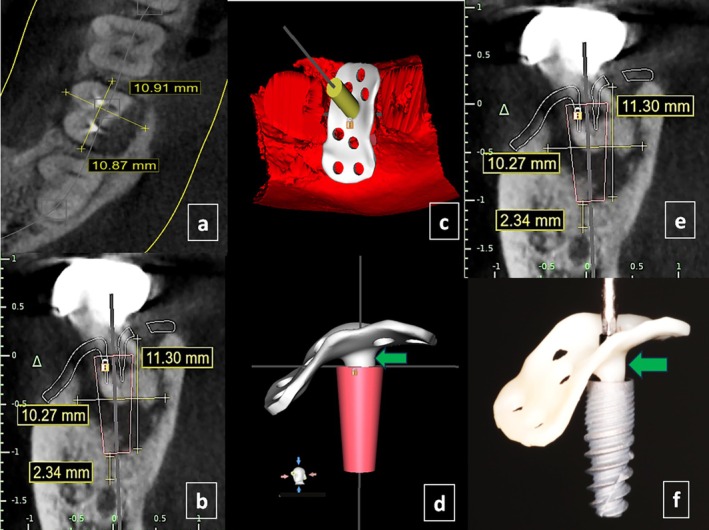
Digital planning of the implant and CZM in the mandible. (a, b) CBCT measures; (c) ZM in white color and axis of the future abutment in yellow; (d) connection of the implant (red) to the ZM connector at the inner surface (green arrow); (e) position of the assembly ZM/Implant; (f) ZM with its connector for clinical use.
